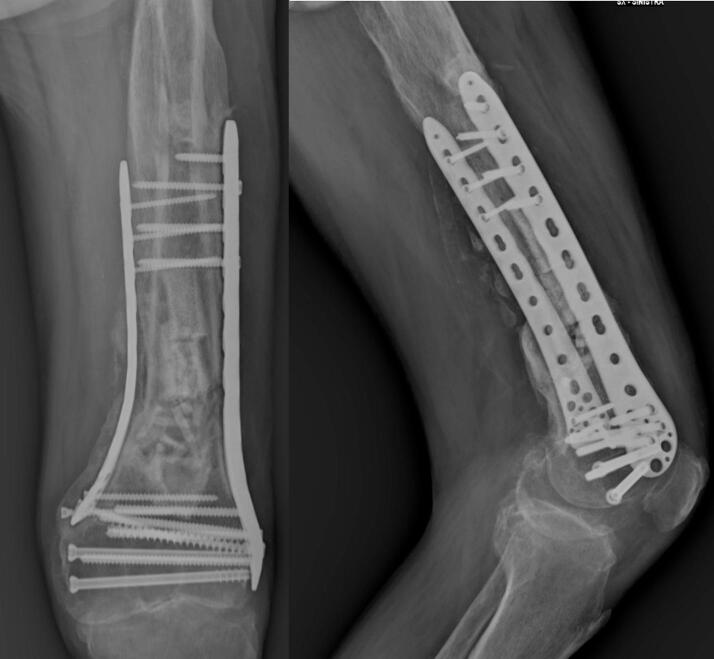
Follow-up X-ray approximately one year after surgery.
